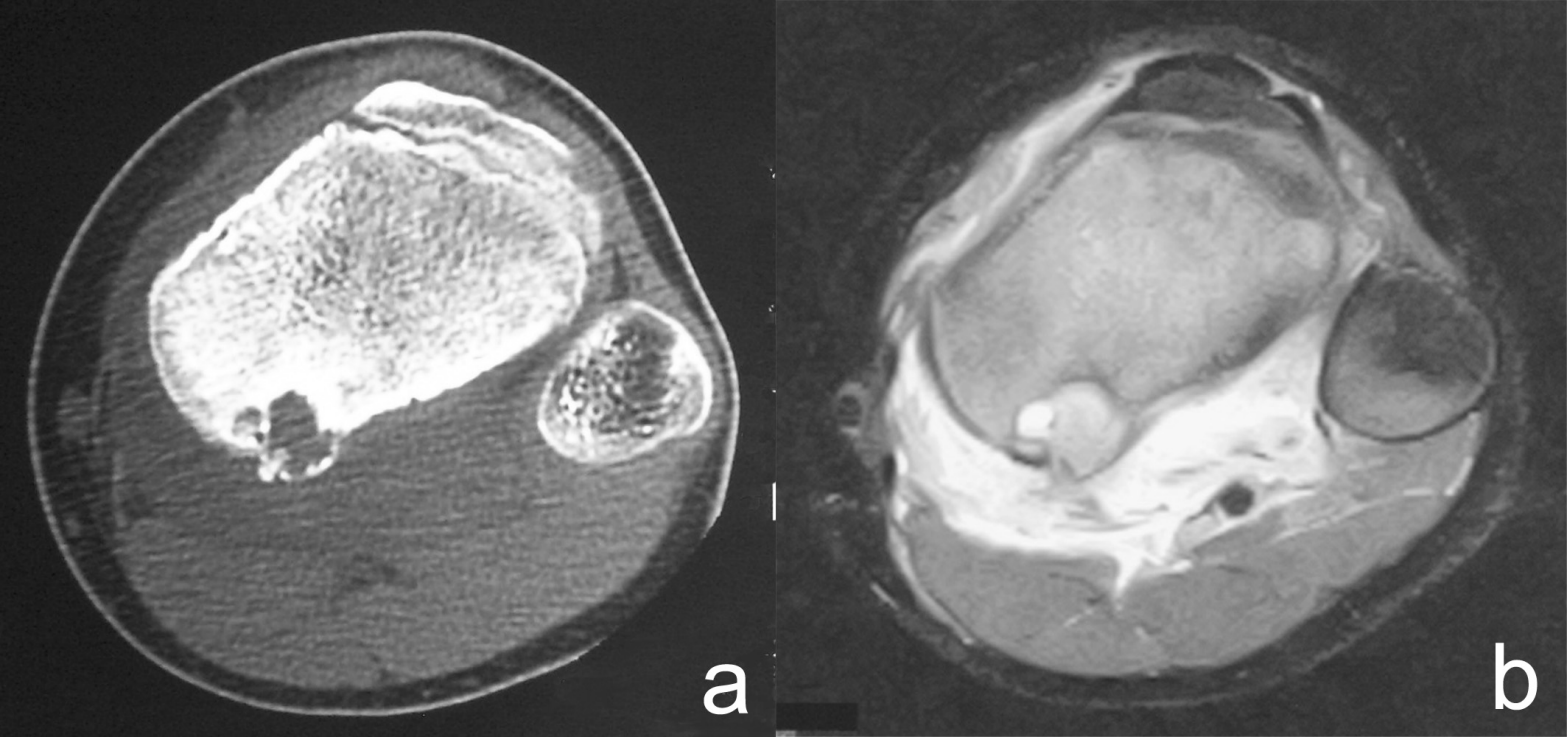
A 13-year-old boy with a solid ABC in the left tibia. a. CT showed an osteolytic expansive lesion with cortical thinning. b. The bony lesion showed a mixture of low and high signal intensity on T2-weighted MR images with an edematous lesion adjacent to the bony lesion.
